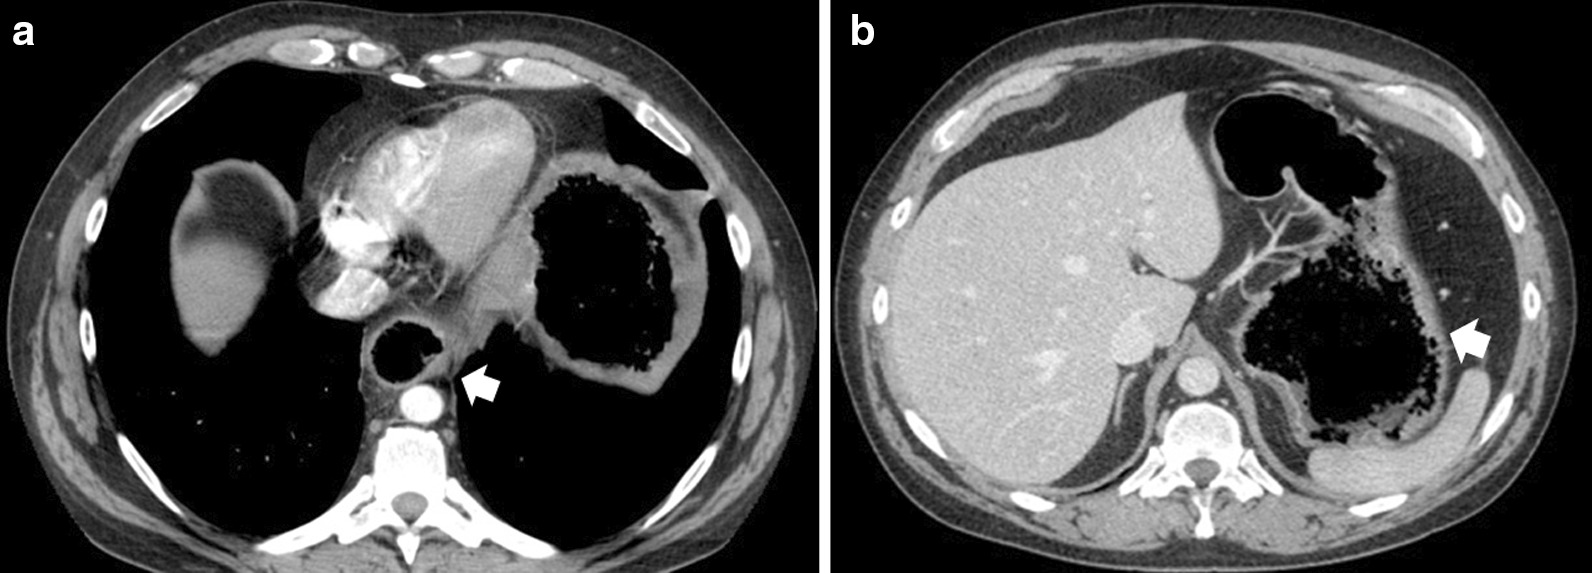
Computed tomography scans showing distension of the esophagus (a) and gas-like–filled bottom of the stomach (a, b)
